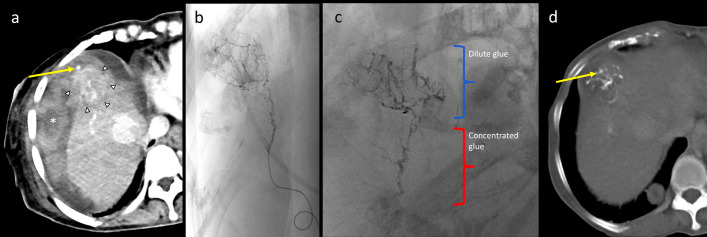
Glue embolisation for ruptured hepatocellular carcinoma using the Nitoryu technique. a CT angiogram shows a ruptured hypervascular tumour in segments 8/4A of the liver (arrowheads) with adjacent perihepatic haematoma (*). A culprit pseudoaneurysm is seen at the tumour surface (orange arrow). b Selective angiogram of the segment 8 tumour-feeding artery in a left anterior oblique 50° projection demonstrates tumour enhancement. c Spot image following glue embolisation using the Nitoryu technique in anteroposterior projection. There is deep penetration of dilute glue (1:7) into the tumour vascular bed, with a more concentrated glue cast (1:3) in the proximal tumour-feeding artery. d Follow-up CT scan demonstrating the glue cast within the culprit pseudoaneurysm (orange arrow)
